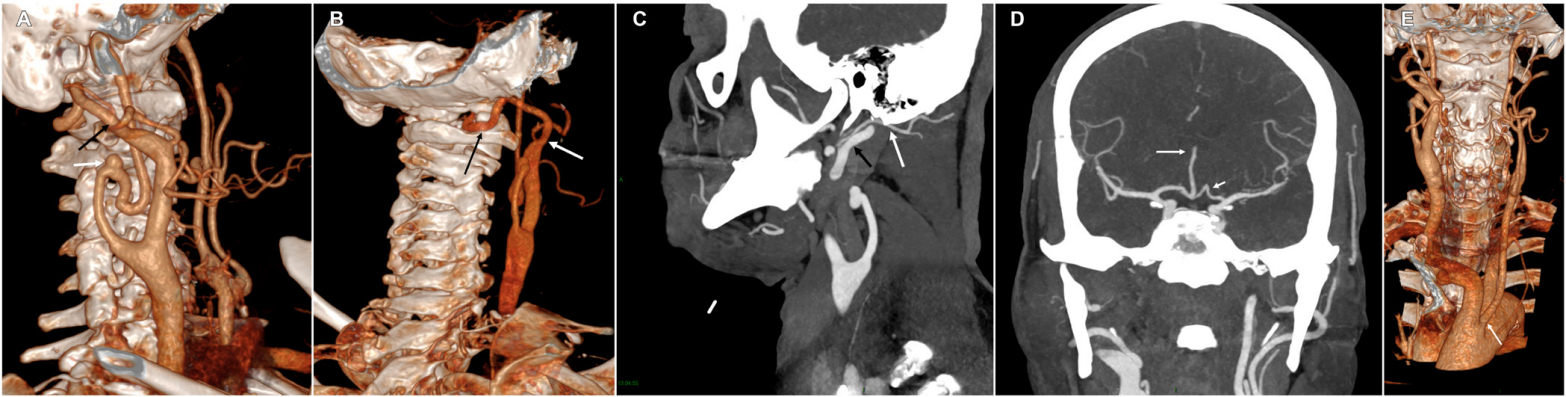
A) Volume-rendering CT angiography shows the type 2 persistent primitive proatlantal artery (PPPA2) arising from a large right external carotid artery (ECA) (black arrow) and a wide-necked saccular aneurysm (5.4 x 6.4 mm in the axial plane) arising from the kinked right internal carotid artery (ICA) at the C3 level (white arrow). B) Volume-rendering CT angiography shows the type 2 persistent primitive proatlantal artery (PPPA2) coursing over the right vertebral artery groove of the posterior arch of C1 (black arrow). Note the origin of the PPPA2 from the right external carotid artery (white arrow). C) Multiplanar reformation (MPR) from the CT angiography shows the right occipital artery (white arrow) arising from the right type 2 persistent primitive proatlantal artery (PPPA2) (black arrow). D) Multiplanar reformation (MPR) from the CT angiography shows an azygos anterior cerebral artery (long arrow) and the kinked A1 segment of the left anterior cerebral artery (short arrow). E) The left anterior oblique projection of volume-rendering CT angiography shows the origin of the left common carotid artery (CCA) that arises from the brachiocephalic trunk (BCT) (arrow).
